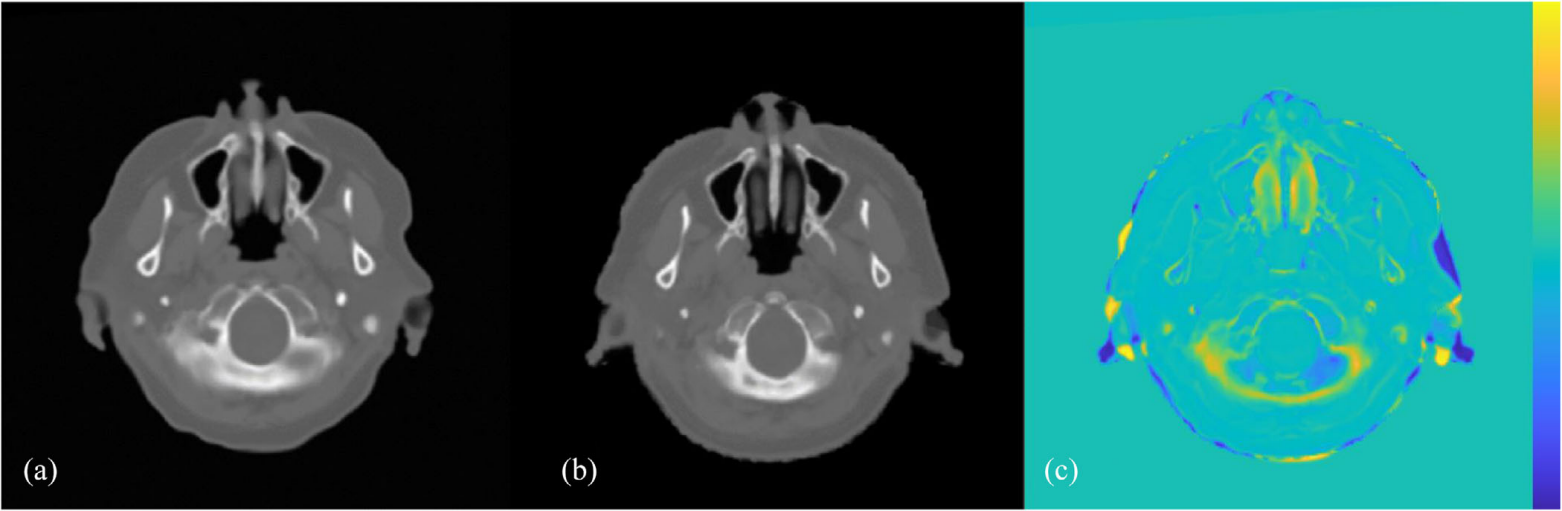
Axial image for sample with highest MAE and RMSE. This showed the effect of facial contour distortion by immobilization device. The resampled MRCAT (a) was subtracted with planning CT (b) to obtain subtraction map (c) with yellow and blue colour contributed from resampled MRCAT and planning CT, respectively.
